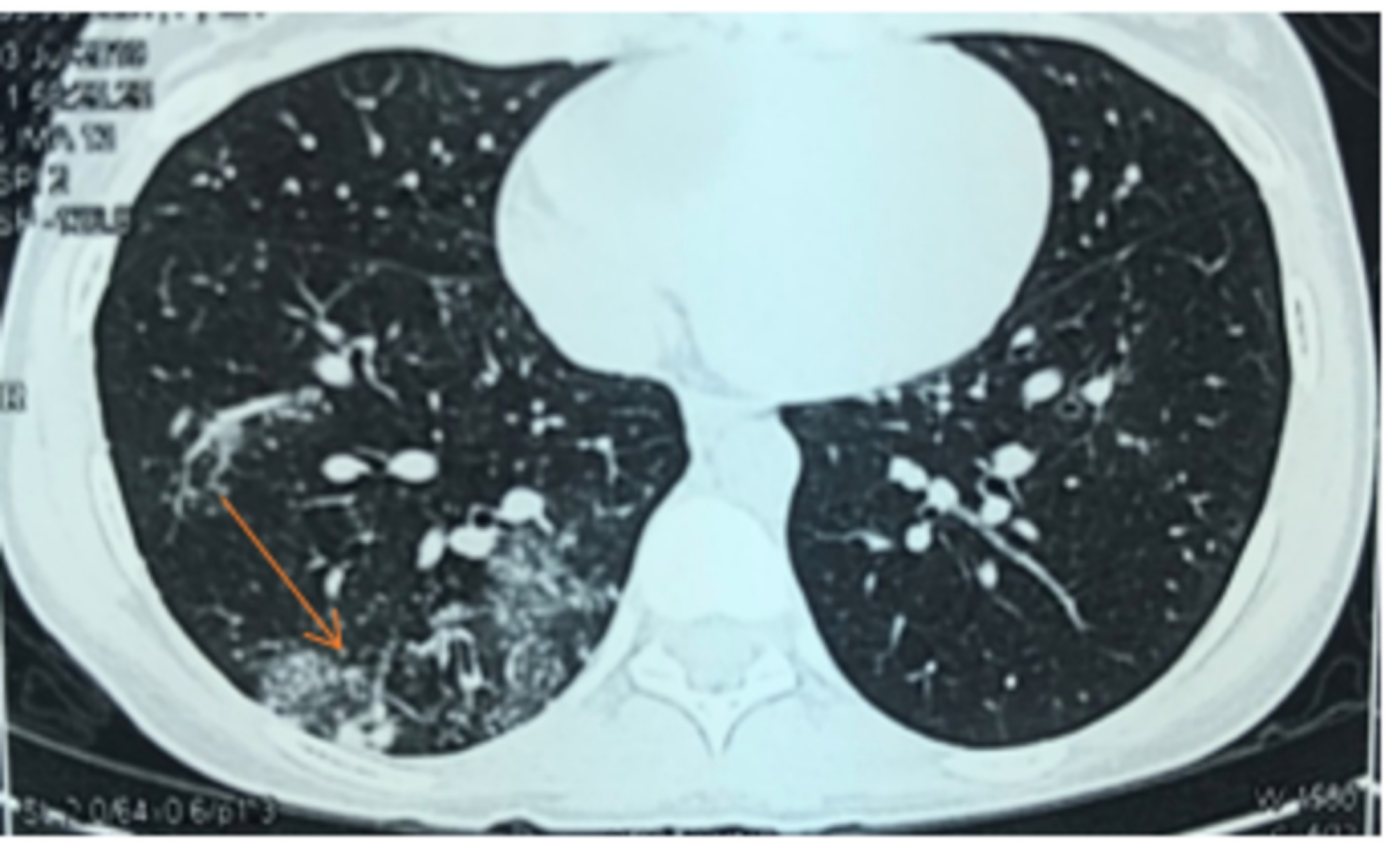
CT scan of the chest shows nodular infiltrates in the right lung with ground glass opacity.
